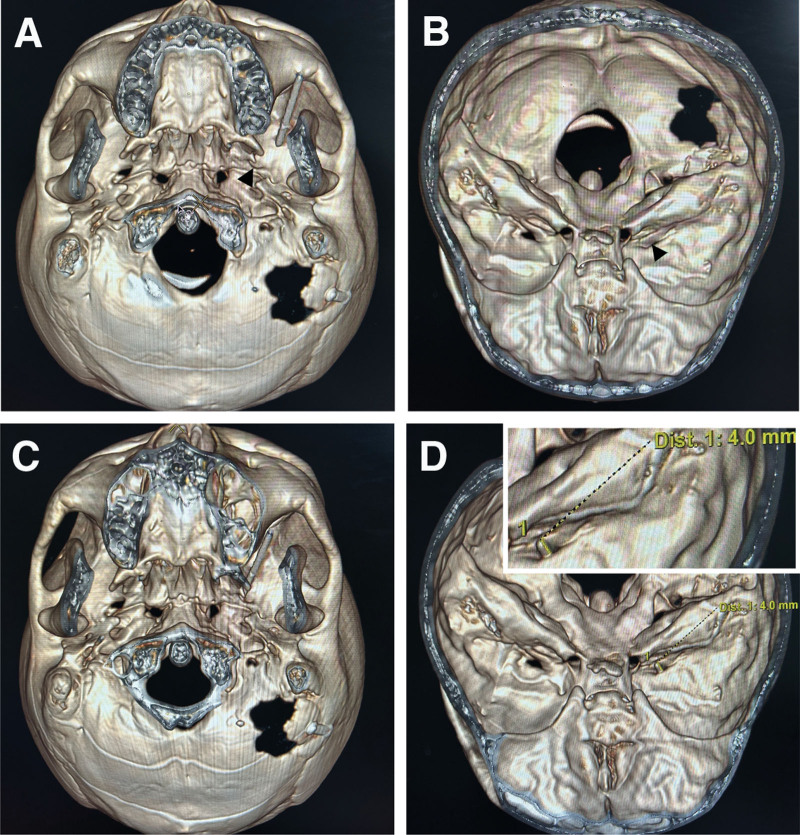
Three-dimensional CT reconstruction imaging showing cannulation of the foramen ovale obstructed by the completely ossified pterygoalar ligament using the Hartel approach in Case 1. (A) Foramen ovale completely obstructed by the pterygoalar bar (black arrowhead) at initial puncture. (B) The black arrowhead indicates the general position of the formed foramen in the interior skull base view. (C) Successful penetration of the cannula into the foramen ovale from the anterior and lateral side of the pterygoalar bar. (D) The position of the cannula tip was approximately 4 mm above the foramen ovale in the interior skull base view. CT = computed tomography.
